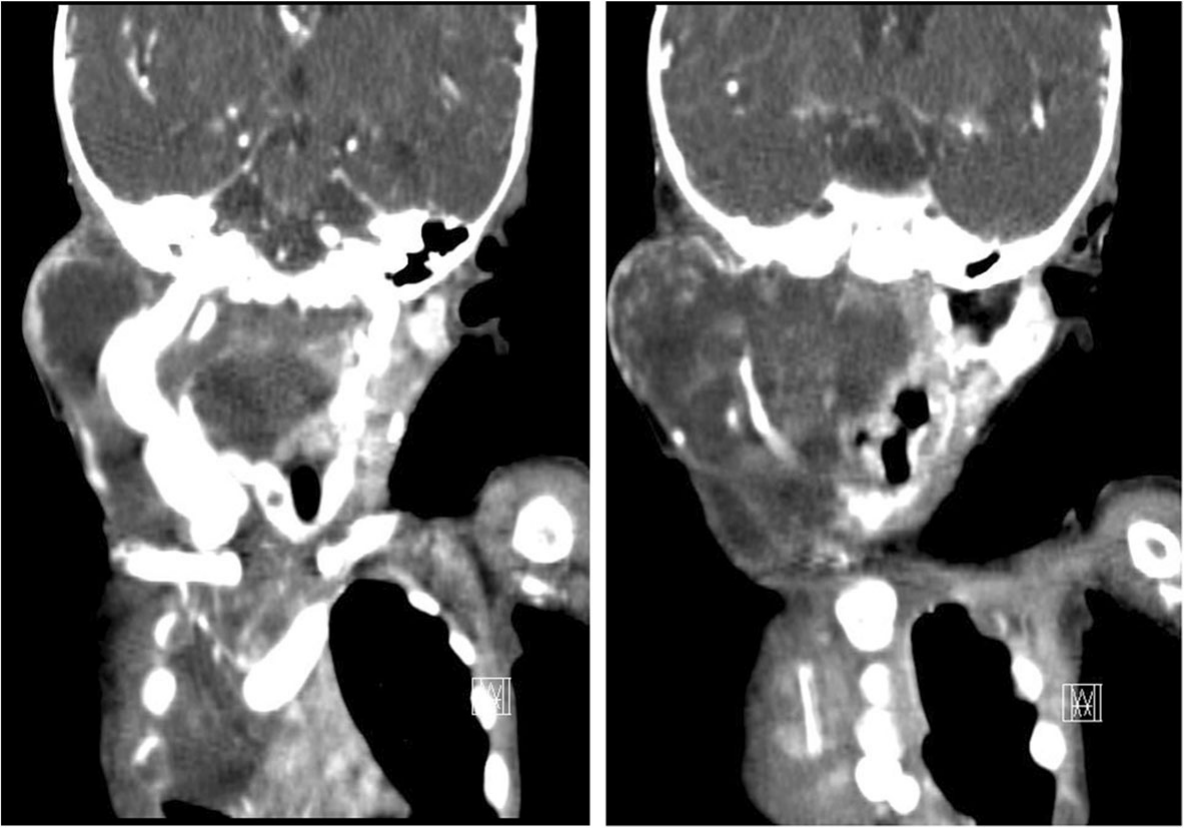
Case 2. Cervico-mediastinal lymphatic malformations after partial excision, visualized on a computed tomography scan
